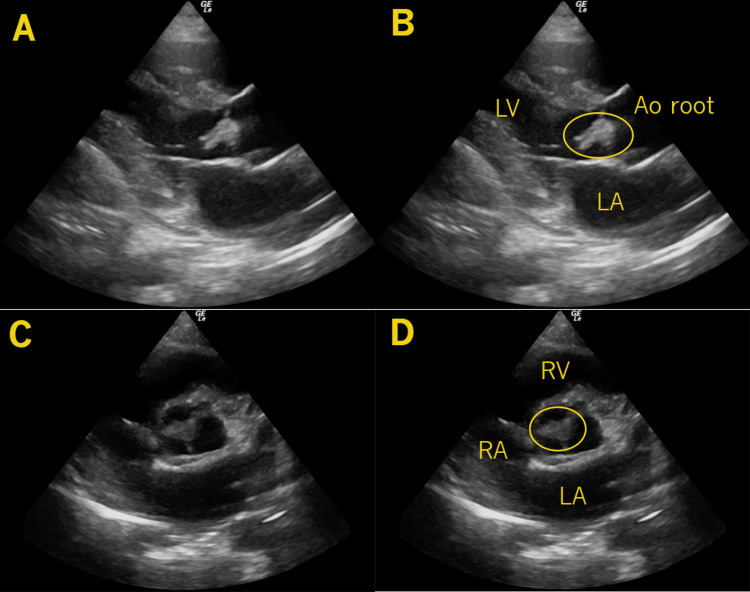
Aortic Valve Vegetation In these images, a large mass (circle) is seen attached to the aortic valve, first from the parasternal long axis view (A-B), where the left atrium (LA), left ventricle (LV), and aortic root (Ao root) are noted. The mass (circle) is also seen from the parasternal short axis view (C-D), where the left atrium (LA), right atrium (RA), and right ventricle (RV) are also noted.
